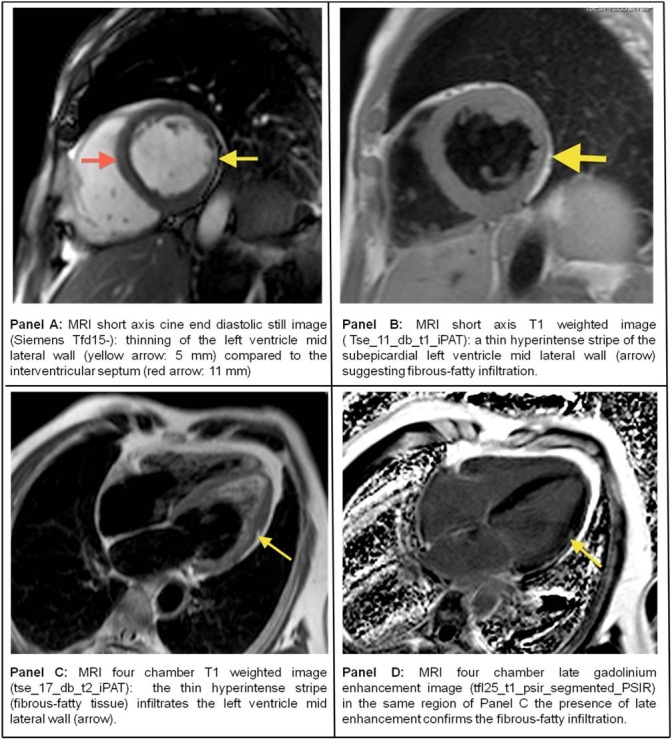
Cardiac MRI images showing morphological alterations in the left ventricle lateral wall (see Panels for details).
